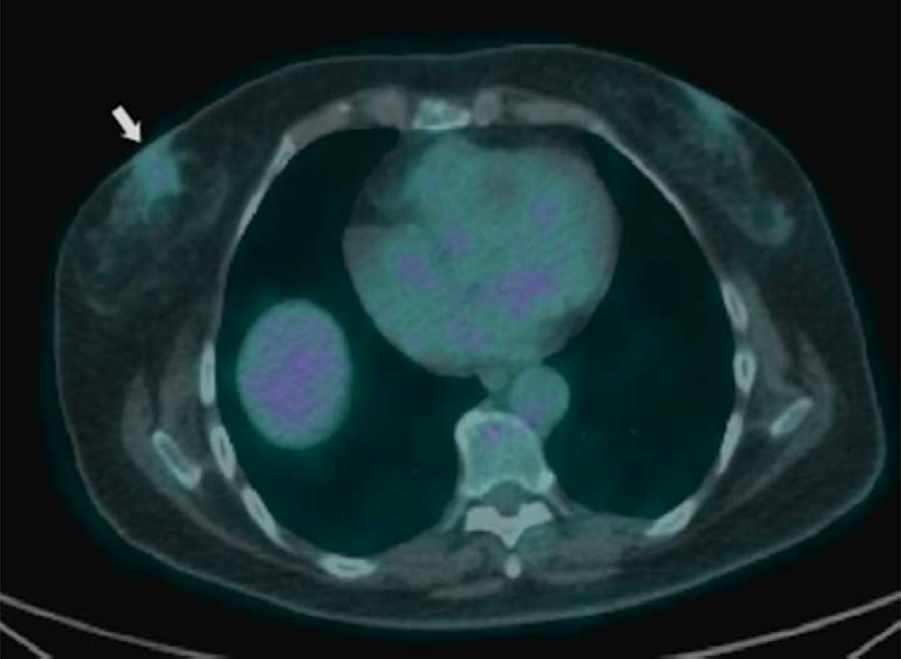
Right retroareolar lesion with mild FDG uptake on PET/CT (white arrow).
